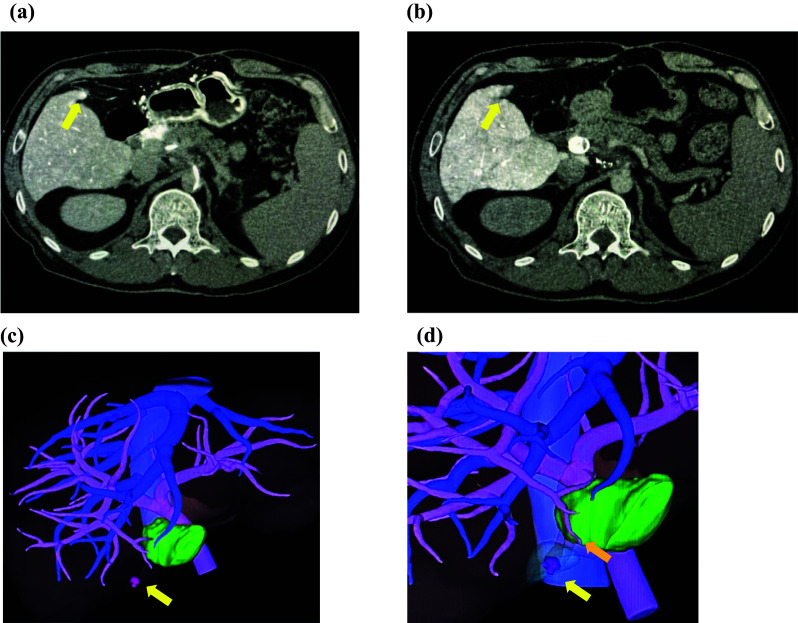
Abdominal computed tomography arteriography and arterial portography. a 8 mm arterially enhanced nodule with corona-like enhancement was observed in segment 5 of the liver (yellow arrowhead). b Same nodule exhibited poor portal blood flow (yellow arrowhead). c Position of the tumor is indicated in the 3D images (yellow arrowhead). d 3D images show that the tumor was located on the edge of segment 5 (yellow arrowhead) and branch of the Glisson 5 (orange arrowhead)
